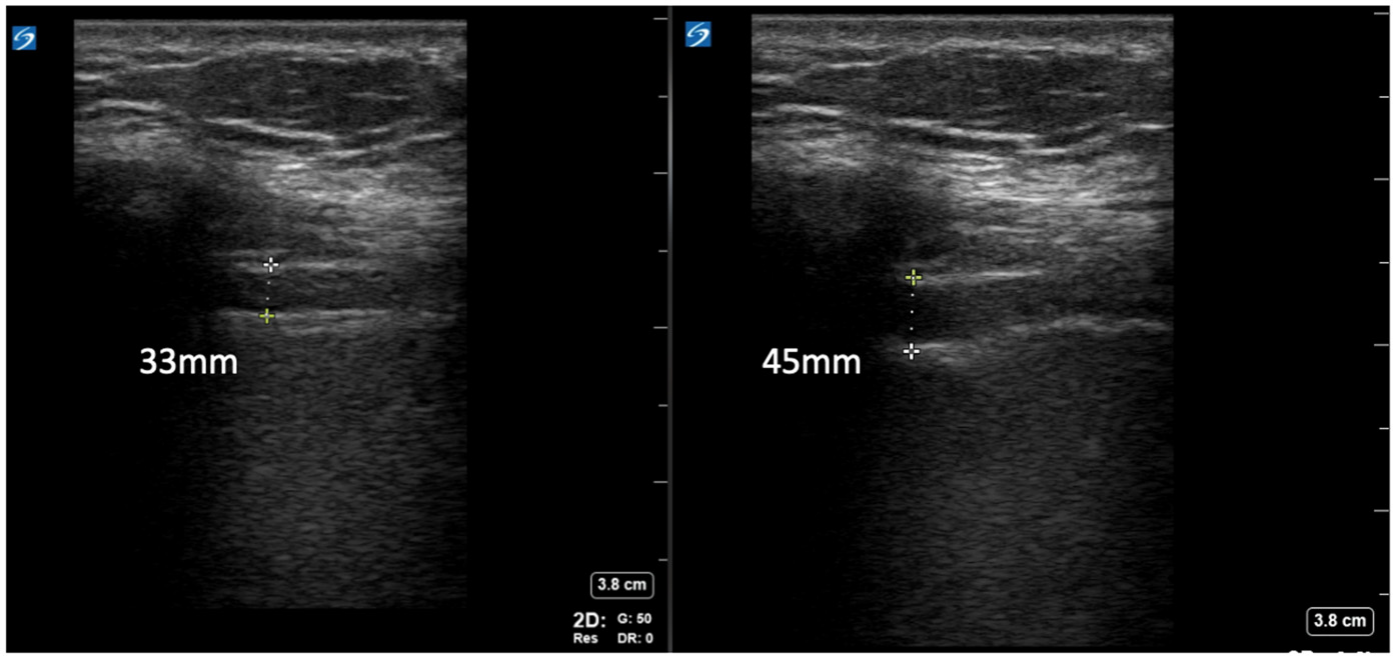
Diaphragm assessment using linear ultrasound probe positioned on the anterior axillary line obtaining B-mode images visualizing diaphragm in: (a) normal expiration and (b) full inspiration. As an example of the measurement of diaphragm parameters – *T*_exp_ = 33 mm, *T*_insp_ = 45 mm, Δ*T* = *T*_insp_  −  *T*_exp_ (45 mm − 33 mm = 12 mm) and ΔTDI% = (*T*_insp_  −  *T*_exp_)*100/*T*_exp_, ((45 − 33)*100/33 = 36.4%). Note that, while M-mode assessment offers easier interpretability and measurements in acquired images, the physical movement of the diaphragm during full inspiration most often would preclude the interpretation of thickness difference in the same spot on the diaphragm, which itself is not of the same thickness in all areas when contracted; using B-mode allows for capturing thickness changes at the same spot, likely improving the accuracy of capturing the thickness changes.
